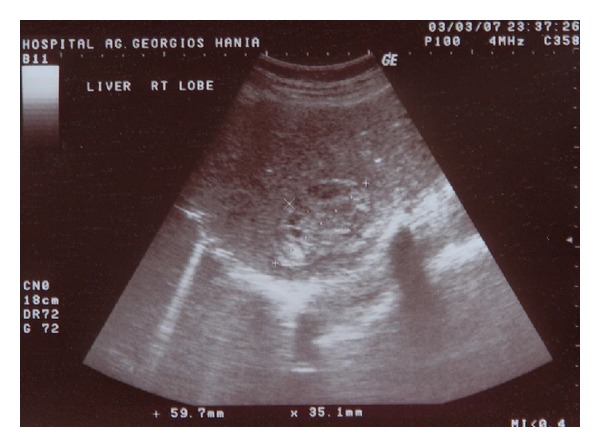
Abdominal ultrasound performed 3 months later reveals further reduction of the hematoma's dimensions.
